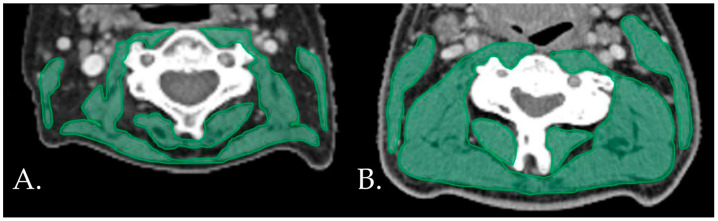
Examples of patients with (A) and without (B) low SMIs on the neck CT.s CT: computed tomography. SMI: skeletal muscle index.
